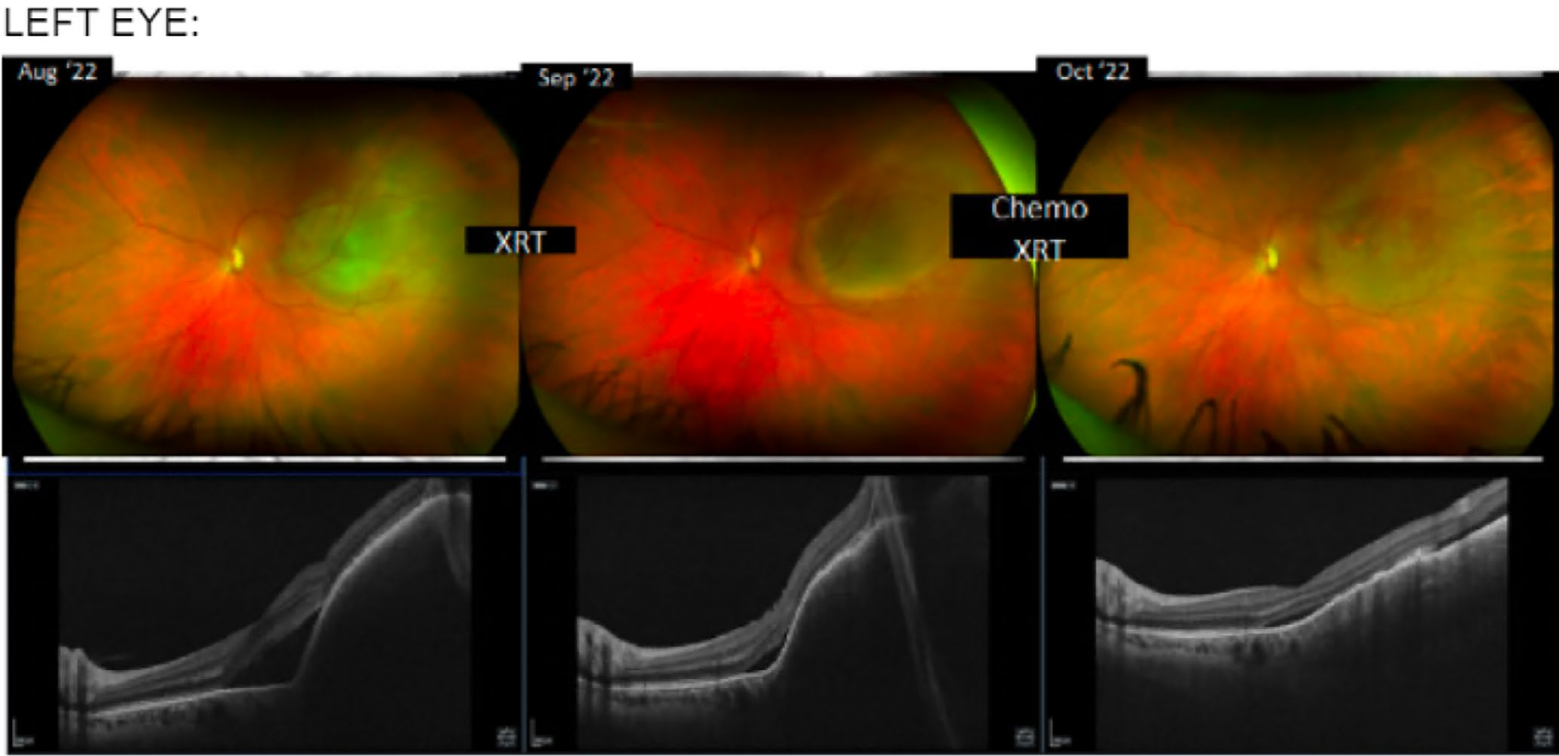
Fundus photography and ocular ultrasound of the left eye demonstrating choroidal metastasis before treatment and dramatic reduction in tumor size with interval development of chorioretinal scar following combination radiotherapy and sacituzumab govitecan.
